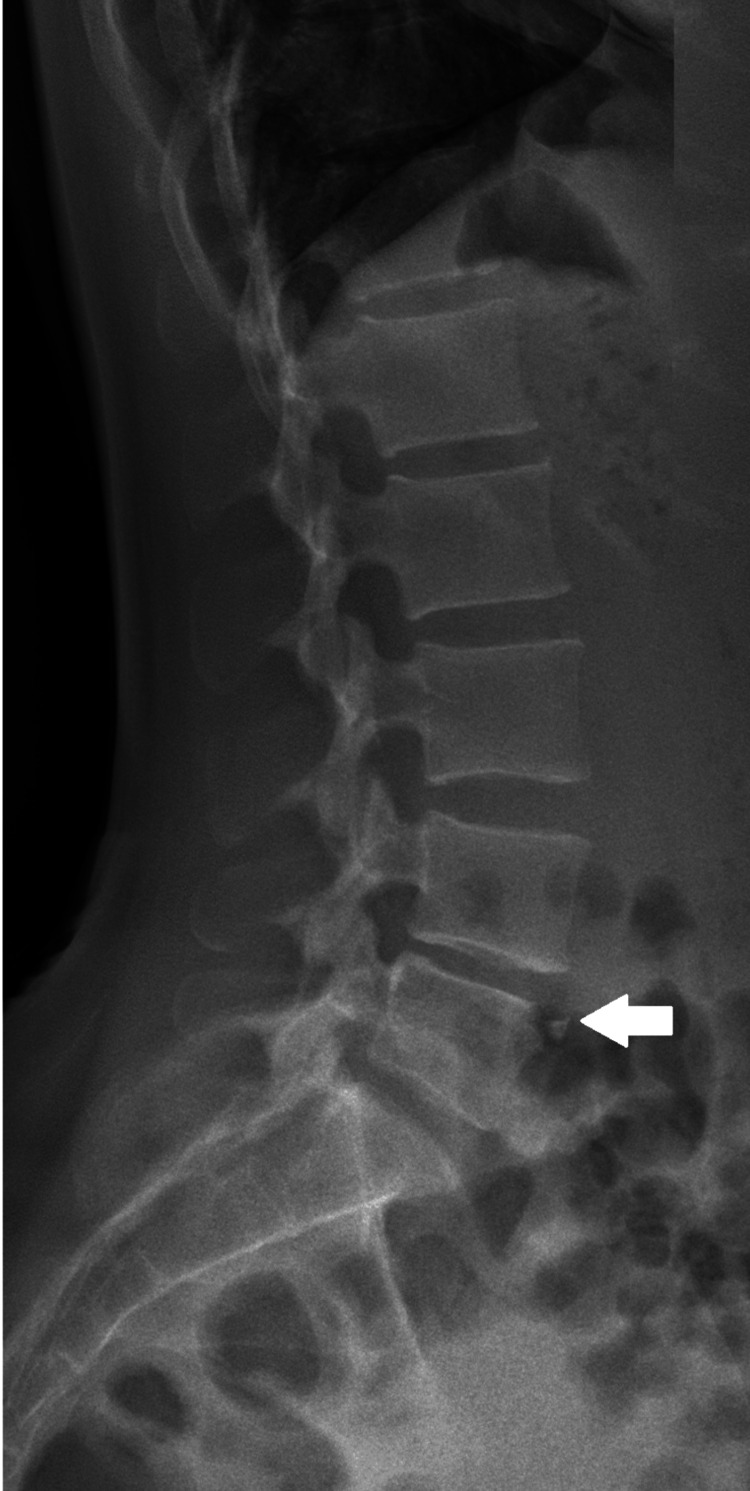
Sagittal plain radiograph of the lumbar spine demonstrating a limbus vertebra Standing lateral lumbar radiograph demonstrated an anterior limbus fragment at the anterosuperior corner of L5. L5/S1 disc height appeared preserved without definite narrowing (white arrow). There was possible mild posterior disc space narrowing at L4/L5 and minimal sclerosis at the posterior superior endplate of L5. No spondylolisthesis was observed.
